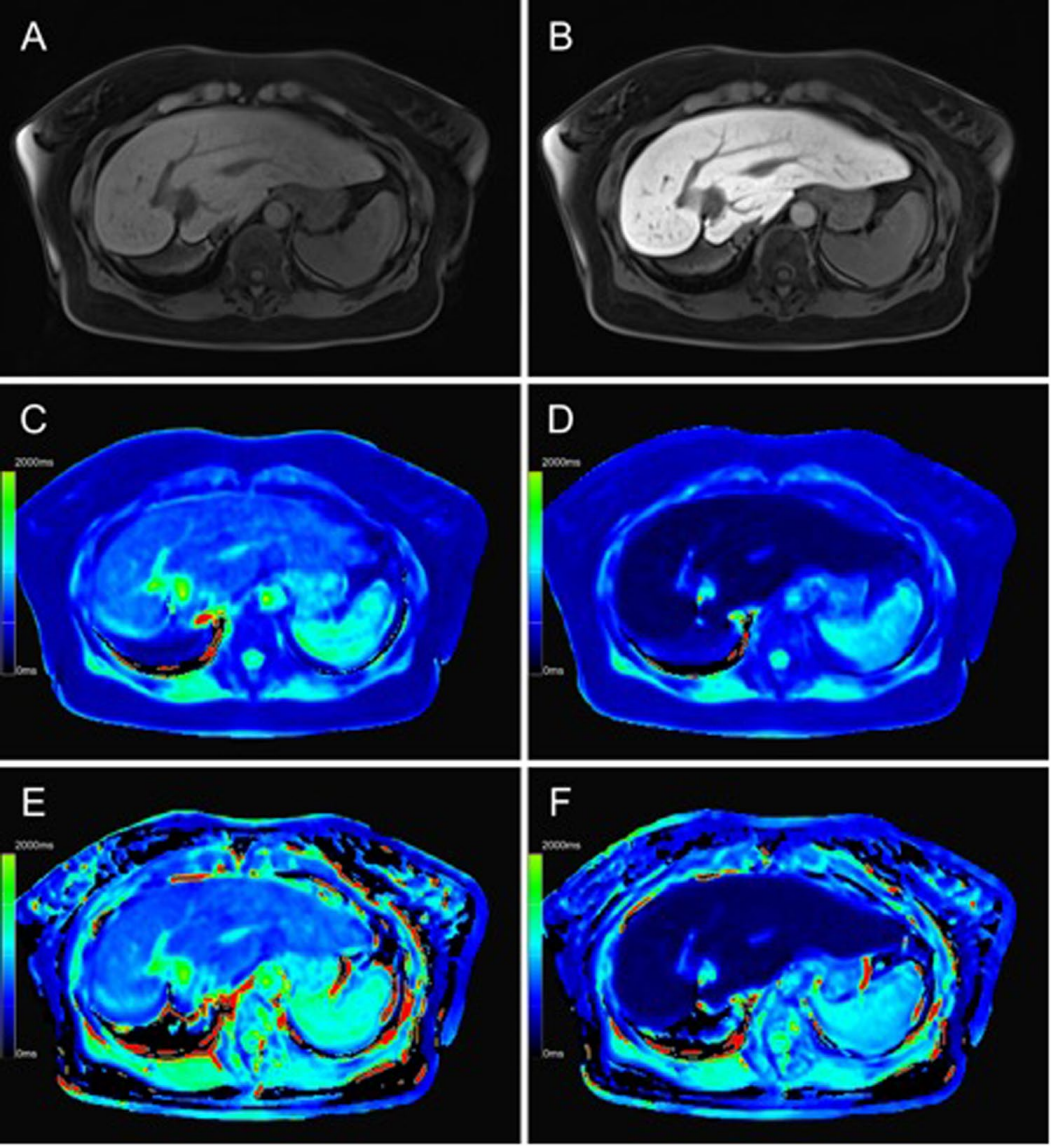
Color-coded T1 maps and T1-weighted sequence based on a 3D variable flip angle sequence of the liver parenchyma of a 47-year-old female patient with regular liver capacity (^13^C-MBT = 327 [µg/kg/h]). T1-weighted VIBE sequence with frequency-selective fat saturation (A,B) and T1 maps (C–F) were generated in native (A,C,E) and hepatobiliary phase (B,D,F). Respective T1-shortening effect can be observed as an increase in signal intensity of the liver in a T1-weighted VIBE sequence (B) and as a dark-blue region in color-coded T1 maps, representing a decreased relaxation time (D,F). Fat-water separation was applied according to the Dixon technique for the T1 maps (E,F). When applying fat suppression on T1 maps (E,F), the overall registered relaxation time increased compared with that of T1 maps without fat separation (C,D). The background of the T1 maps was manually set to black to facilitate color visualization.
